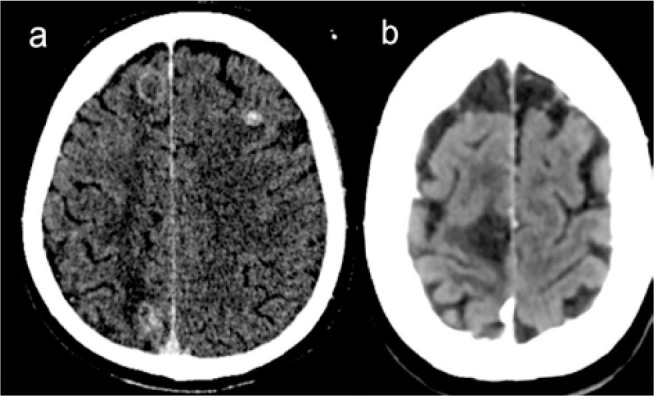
Contrast-enhanced computed tomography. A: Hypodense
